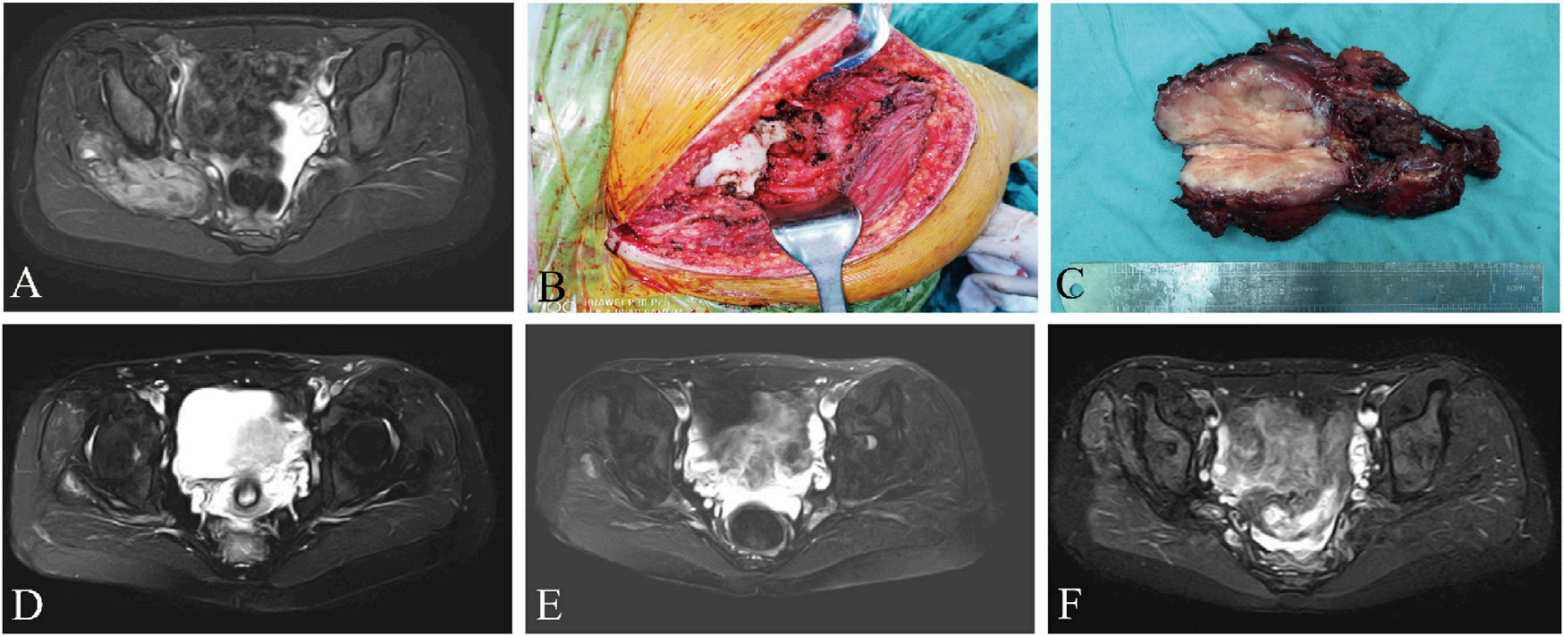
A 21-year-old female presented with DF of the right buttock, preoperative MRI (A), surgical resection of the tumor (B, C), and reexamination of MRI (D–F) after 27, 39, and 49 months after surgery, respectively. MRI, magnetic resonance imaging.
